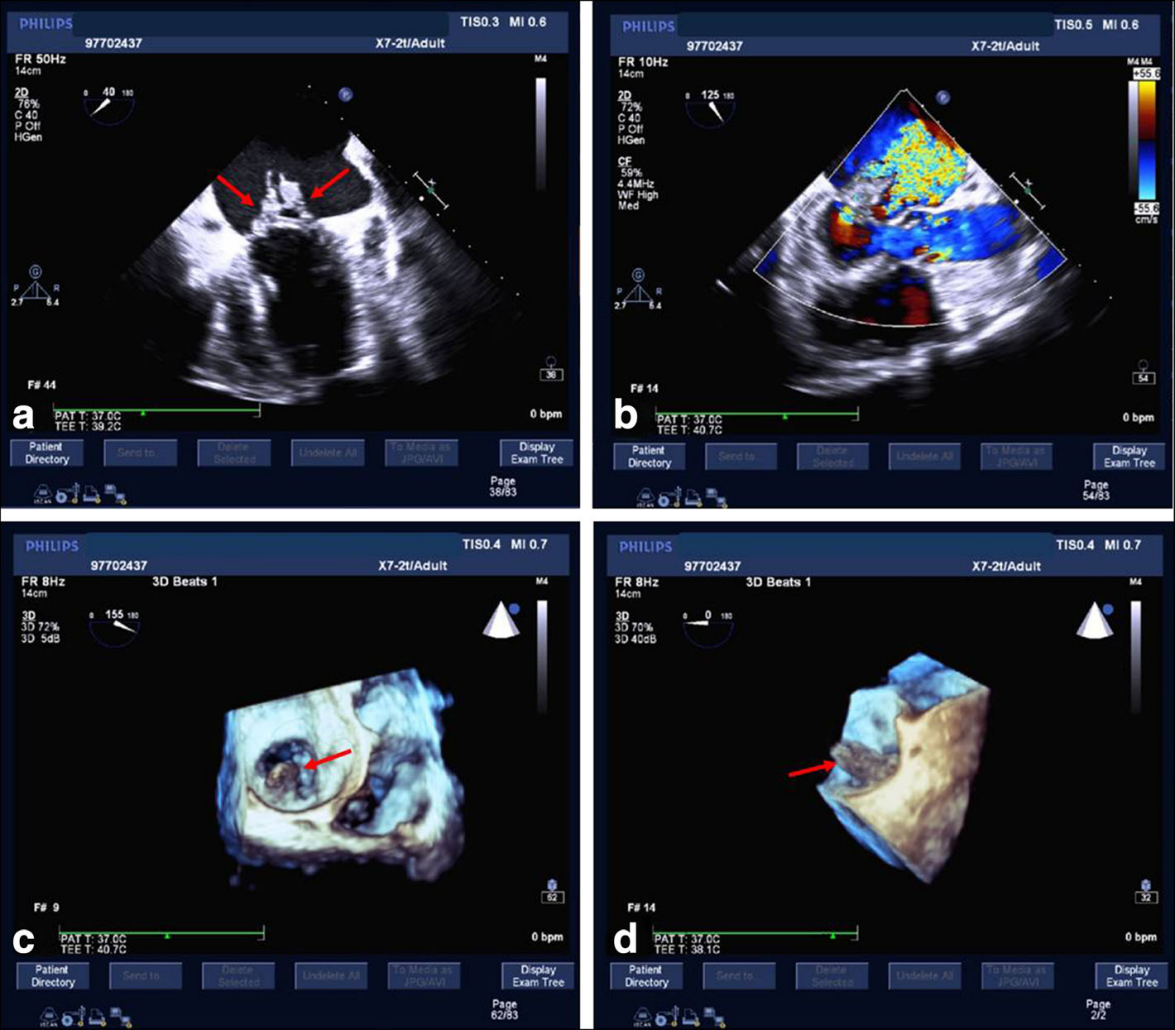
Images of mitral valve by 2D and real-time 3D transesophageal echocardiography (TEE). a Medium to high-echoic vegetations adhering to anterior and posterior mitral leaflets and part of the posterior mitral leaflet prolapsing into left atrium in systole by 2D TEE. b. Severe mitral insufficiency and perforation with regurgitation; c and d. A bird-eye view and a lateral view of mitral valve vegetations from left atrium by real-time 3D TEE
